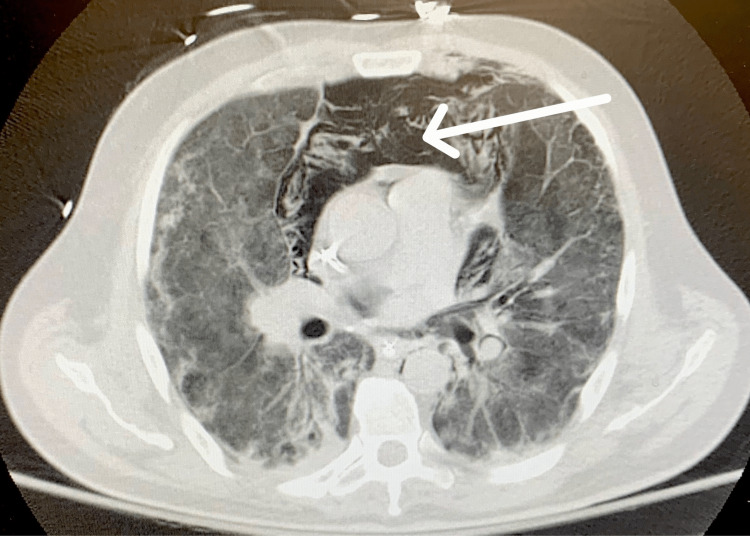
CT scan with an arrow pointing to pneumomediastinum.
